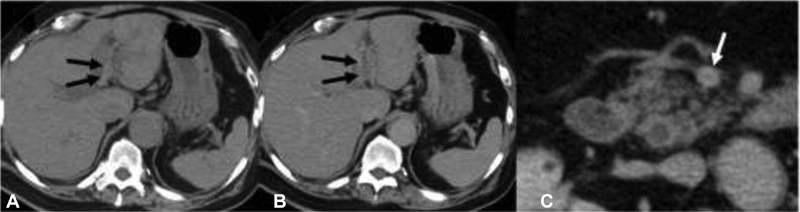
Computed tomography (CT) showing thrombus (
